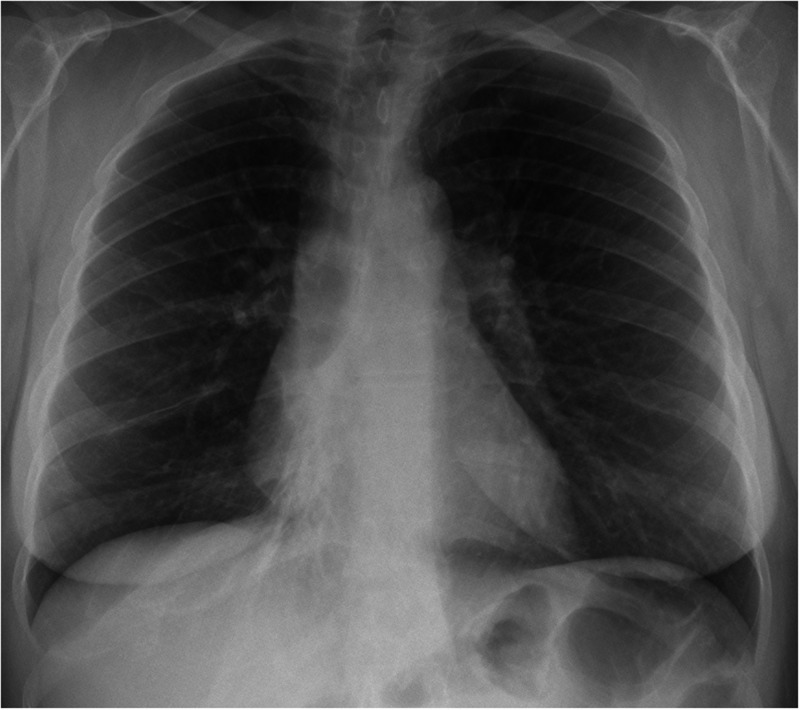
Chest X-ray at postoperative Day 3 showing a well-expanded right lung with only minor residual atelectasis at the base.
